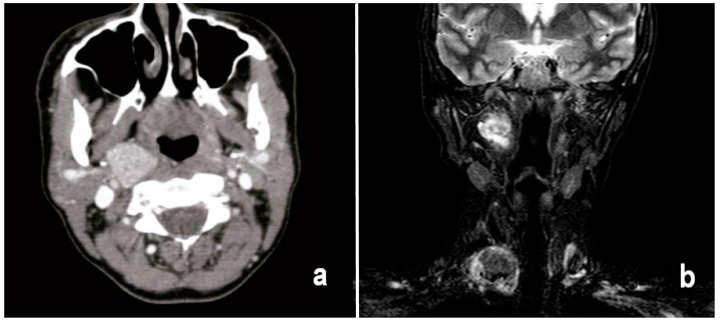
(a) Axial CT scan of the parapharyngeal node; (b) coronal MRI scan showing thyroid mass and parapharyngeal metastatic node.
